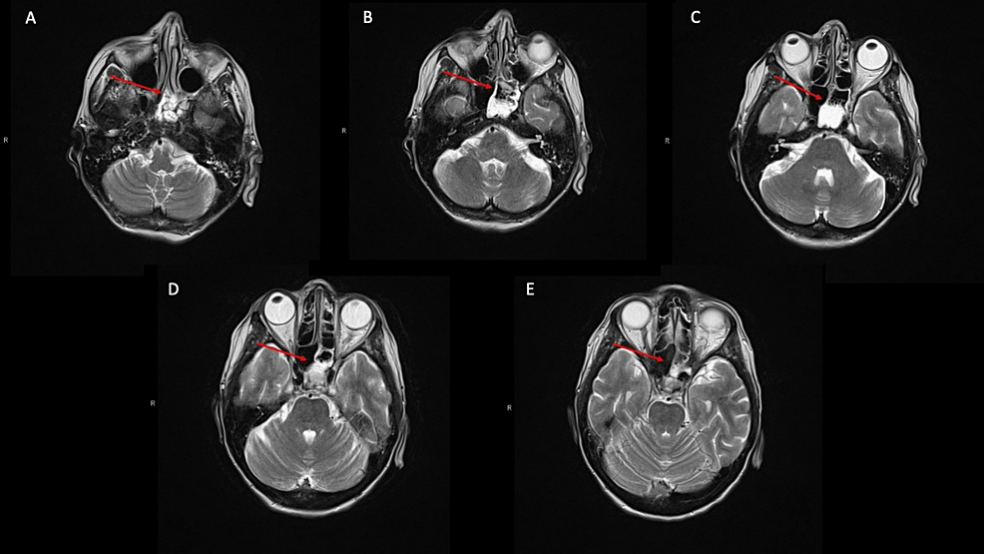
Brain MRI Red arrows denote the abnormal findings. A-E represent the image sequence flow, moving from inferior to superior. Radiology report described no acute intracranial or intraorbital abnormalities seen. Opacification of the left sphenoid sinus consistent with acute sphenoid sinusitis. Minimal small vessel ischemic change and tiny old left cerebellar lacunar infarct. Partial opacification of the left mastoid and minimal opacification of the right mastoid. No thrombosis of a cavernous sinus. Orbits are normal. No compression of the optic chiasm. Thin sections through the orbits demonstrate optic nerves, extraocular muscles, and intraorbital fat to be within normal limits and not compressed.
